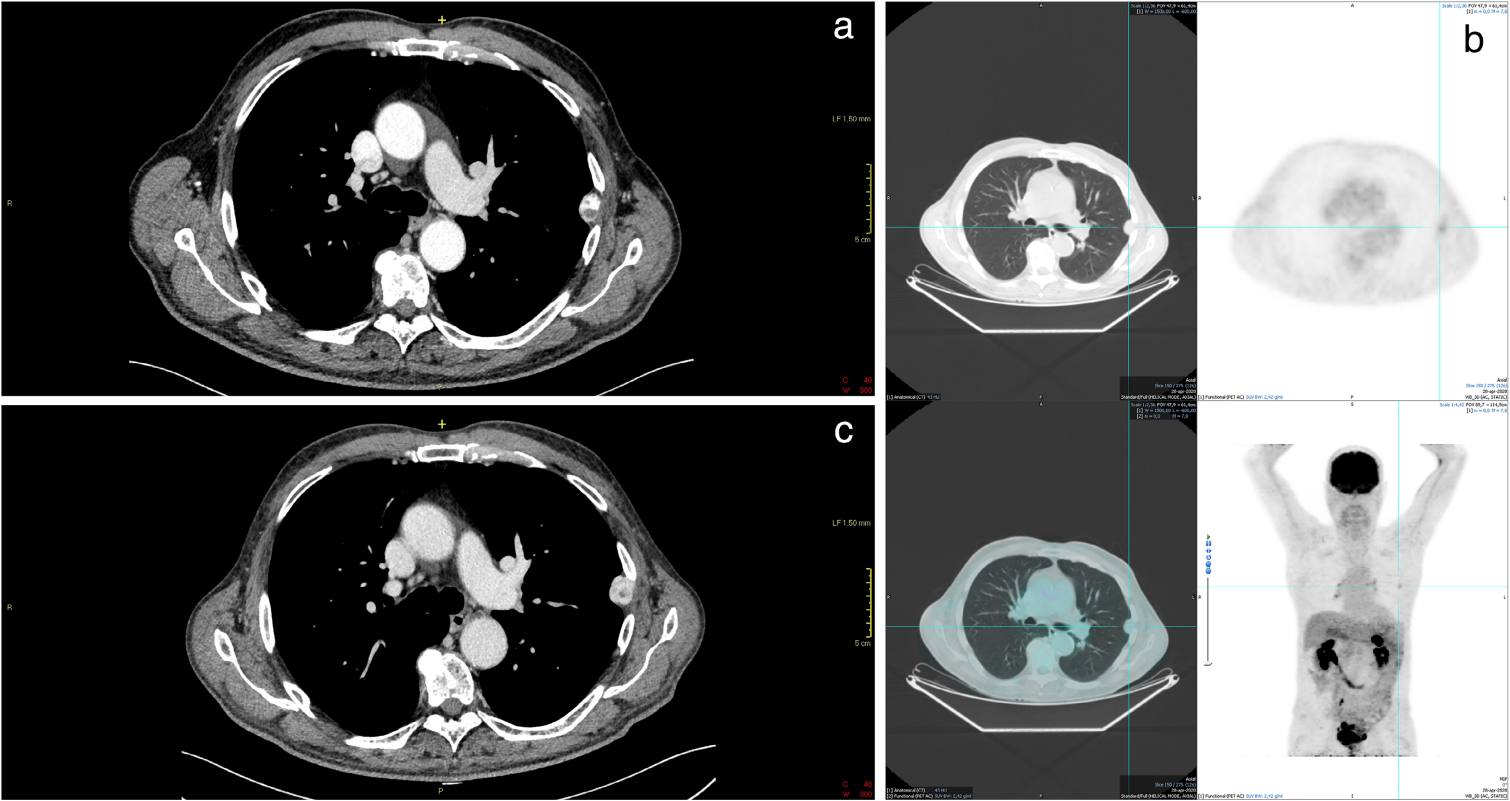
(a) Axial CT scan showing subpleural lesion of ~19 mm in size with regular margins and high contrast enhancement, arising from the thorax wall. (b) 18(F)‐fluorodeoxyglucose (FDG) positron emission tomography (PET) demonstrating a mild FDG uptake (SUVmax 2.4) of the thoracic lesion. (c) One‐year‐after axial CT scan showing the known subpleural lesion increased in size (24 × 22 mm), highly vascularized without signs of infiltration
